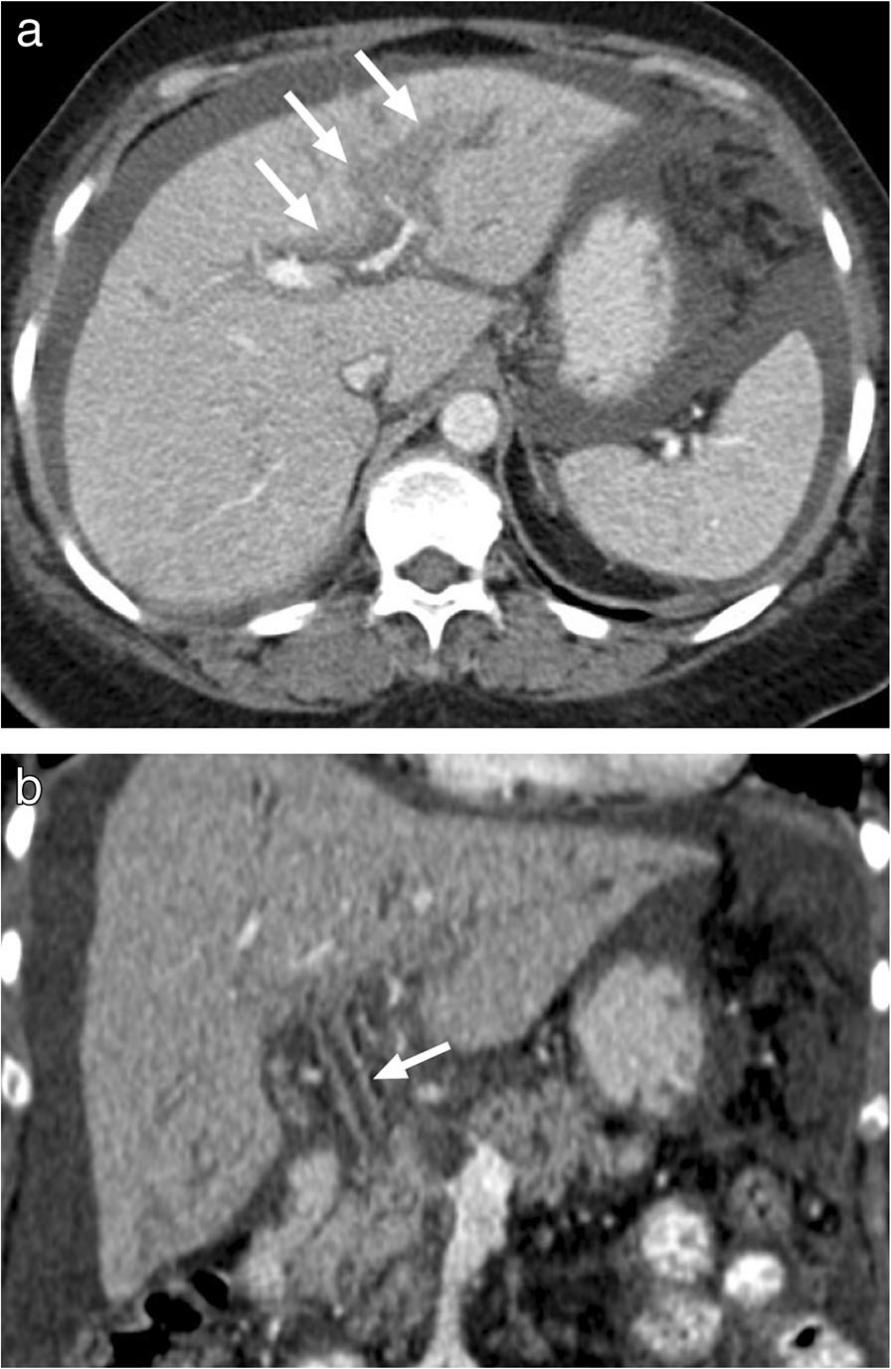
A 68-year-old female with known remote history of breast cancer. The patient recently started to experience abdominal pain, distention, night sweets and weight loss. a Axial plane postcontrast CT demonstrated prominent periportal thickening (arrows) in the left liver lobe which may also be seen in infiltrative liver malignancies such as lymphoma. b Coronal reformatted image shows significant contrast enhancement in the common bile duct (arrow). Also note moderate ascites with mild peritoneal contrast enhancement which may also suggest peritoneal carcinomatosis. Intraabdominal fluid sampling and cytology confirmed biliary TB
